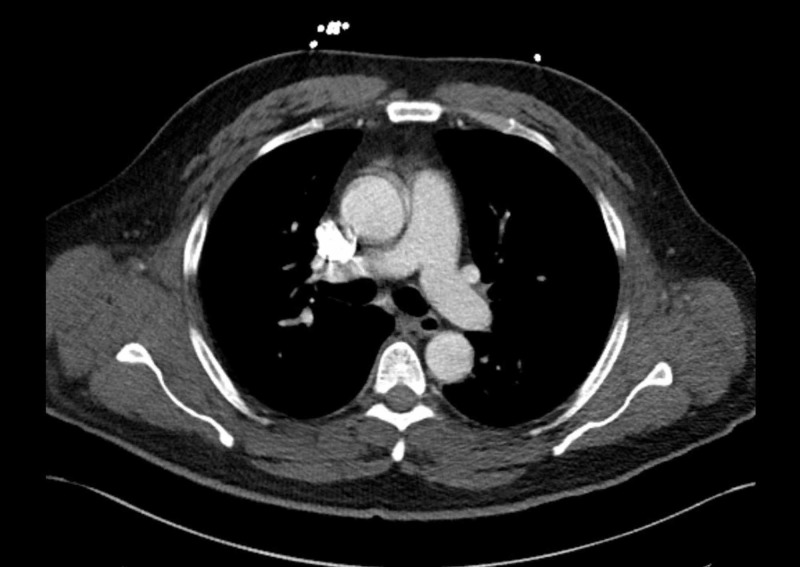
CT angiogram of the chest The image shows no evidence of pulmonary embolism from the main pulmonary artery through the bifurcation to the right and left pulmonary arteries CT: computed tomography
